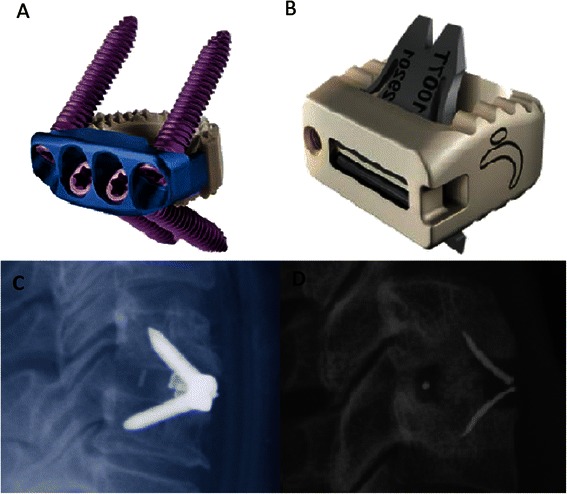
Low Profile Integrated Plating. A) Zero-P cervical cage V B) ROI-C cervical cage C) Radiograph demonstrating Zero-P placement D) Radiograph demonstrating ROI-C placement.
